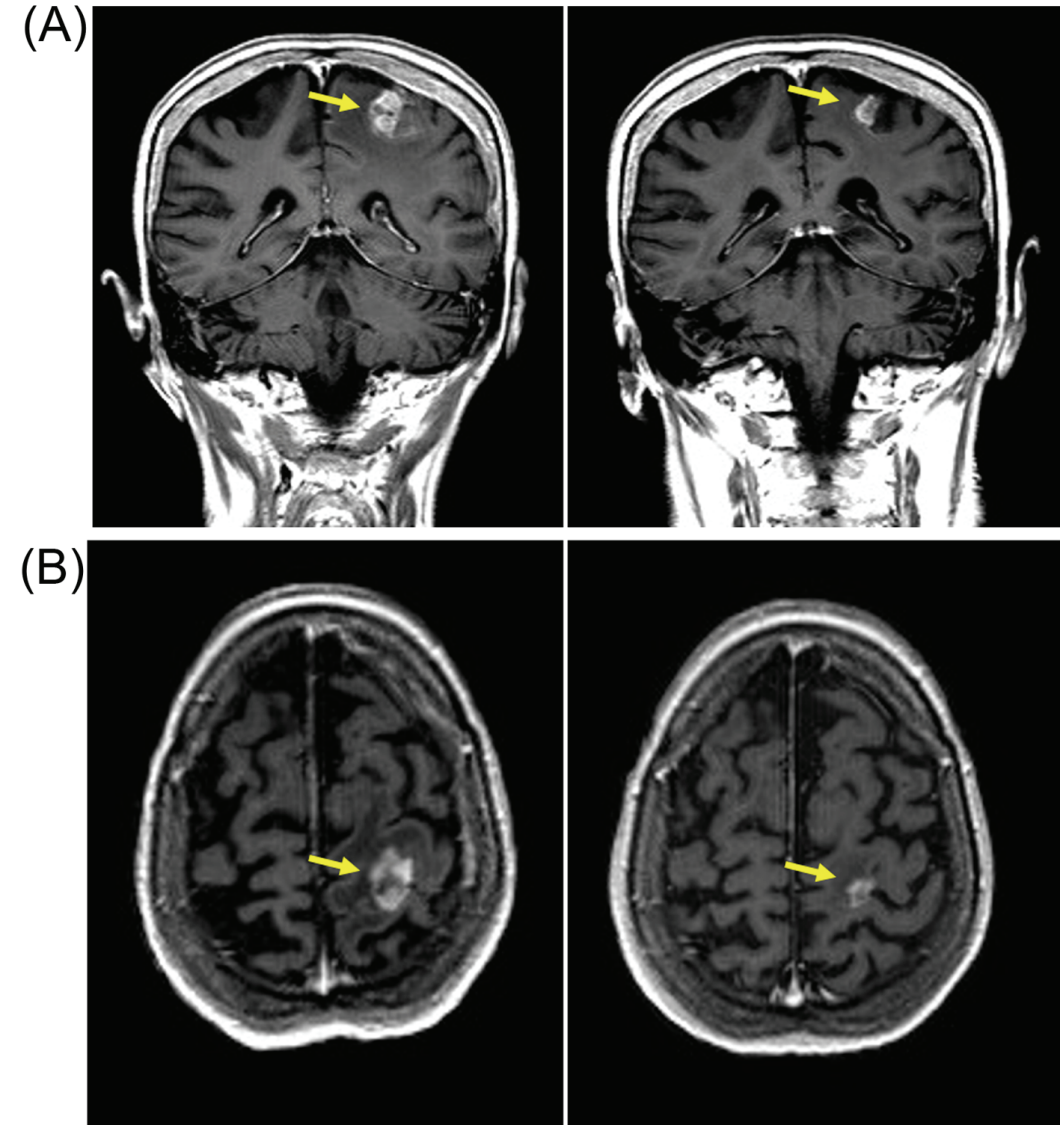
Patient developed response after re-challenge schedule of reduced-dose pazopanib (600 mg/day) with reduction in size of left parietal brain metastasis. (A) Coronal T1-weighted scan with gadolinium contrast demonstrating contrast enhancing lesions (yellow arrow) on baseline MRI scan (left panel) in left parietal region that resolved after 3 months of pazopanib (right panel). (B) Axial T1-weighted baseline MRI scan with gadolinium showing left parietal metastasis (left panel) that resolved after 3 months of pazopanib (right panel).
